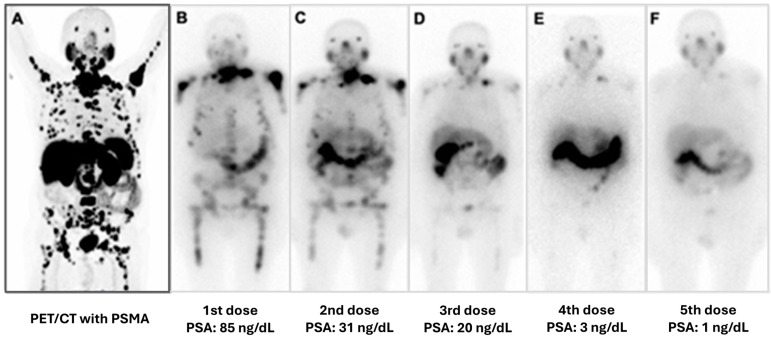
Example of the Theranostic concept (molecular radiolabeling for diagnostic and therapy purposes). In this case, the same molecule (PSMA) was radiolabeled with two different isotopes (gallium-68 and lutetium-177). ^68^Ga-PSMA was used for diagnosis of the extent of metastases from prostate cancer to plan therapy with ^177^Lu-PSMA (A) ^68^Ga-PSMA PET/CT image of a patient with extensive, wide-spread bone metastases from advanced prostate cancer with high PSMA uptake. Because of the avidity of the cancer cells to PSMA, the patient was programmed for personalized therapy with ^177^Lu-PSMA. (B–F) images obtained 4 h after therapy showing the extent of bone metastasis with an initial PSA level of 85 ng/dL. In these subsequent cycles, the images obtained show excellent response to treatment. The patient presented with a progressive reduction of PSA levels, improvement of symptoms and performance status, and significant reduction of bone metastasis.
